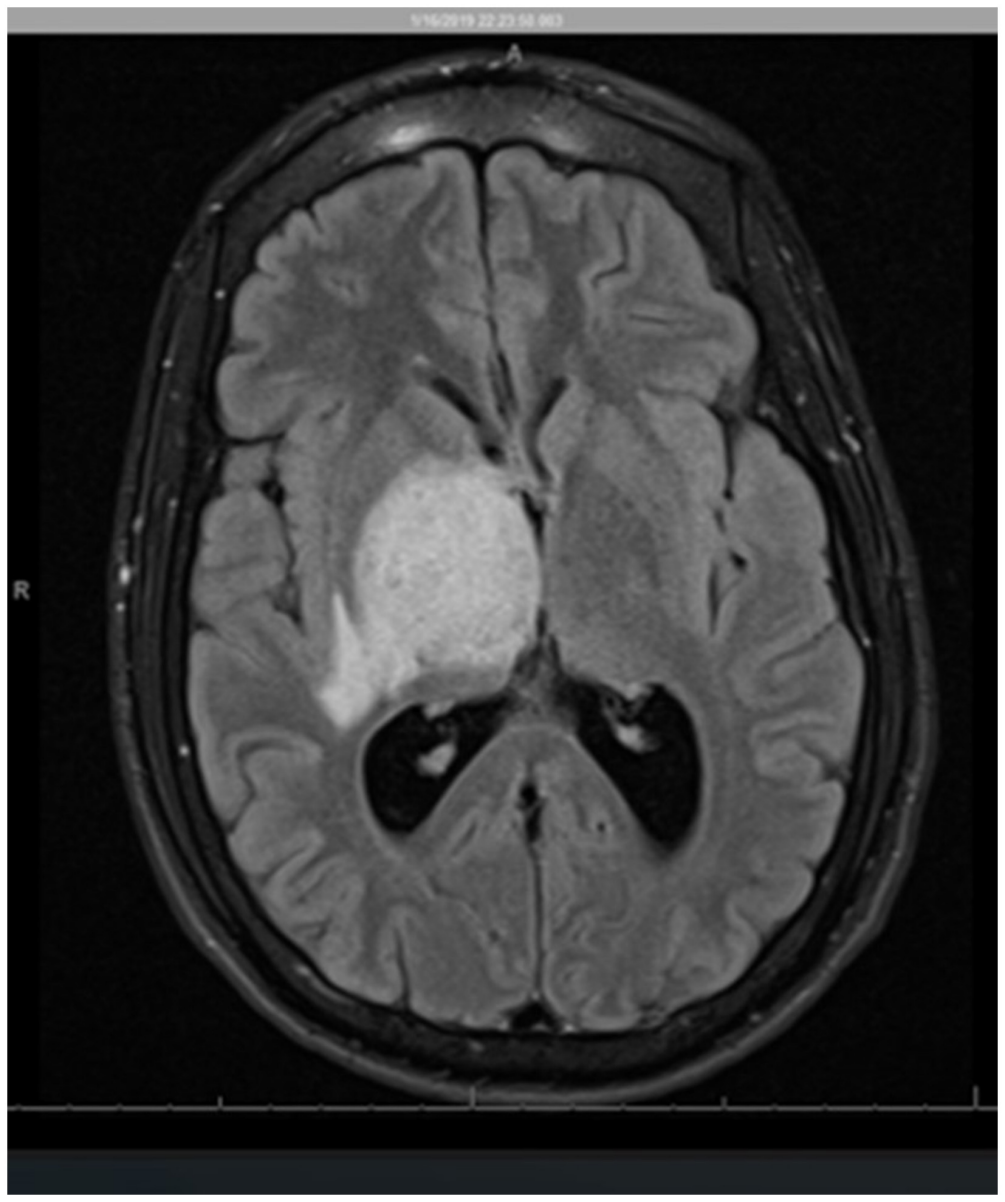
Axial view MR imaging (T2 FLAIR). The image shows a heterogeneous region centered in the right thalamus/gangliocapsular region, involving the entire right basal ganglia, including the putamen, globus pallidus, caudate, and the posterior limb of the internal capsule as well as the posterior aspect of the external capsule. There is a 1-cm right-to-left midline shift and compression of the lateral ventricles and the third ventricle with subsequent mild dilatation of the lateral ventricles concerning obstructive hydrocephalus.
